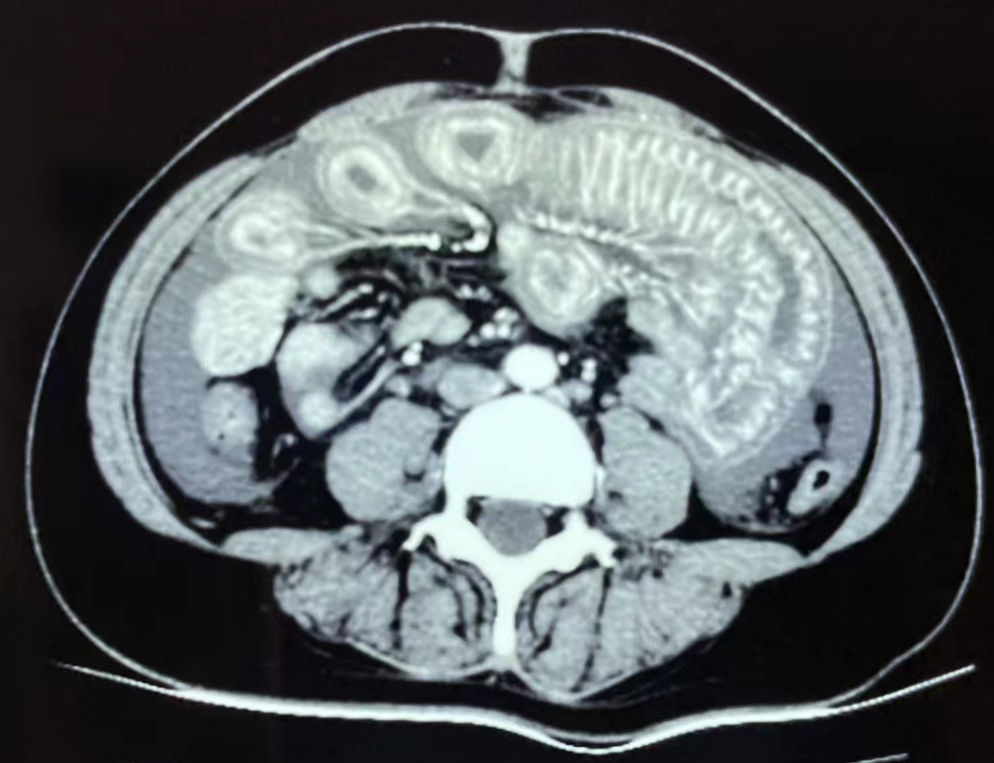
A computed tomography (CT) scan showed changes in the mesenteric vessels, dilated bowel loops, thickened bowel walls, and the “double-halo” or “target” sign before the start of treatment.
